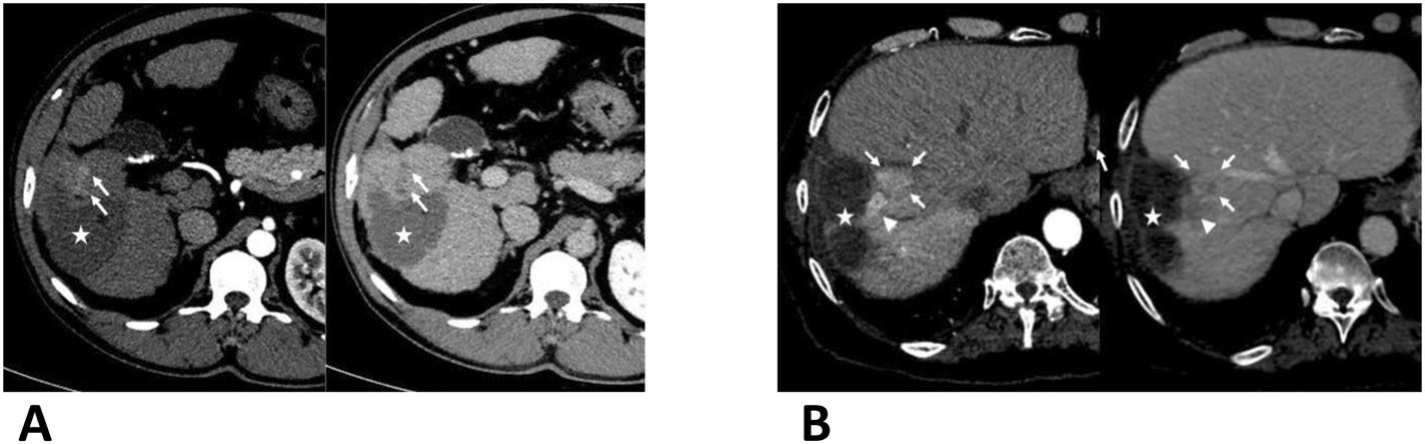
Imaging features of aggressive intrasegmental recurrences. Axial CT scans showing the two patterns of aggressive intrasegmental recurrences (arrows) adjacent to ablation zone (stars), at arterial (left parts) and equilibrium phases (right parts), (A) simultaneous development of multiple nodular (at least three, the 3rd nodule is outside the plan of this slice) or (B) infiltrative mass in the treated segment associated with tumoral invasion of segmental and/or sectorial portal branches (arrowheads).
